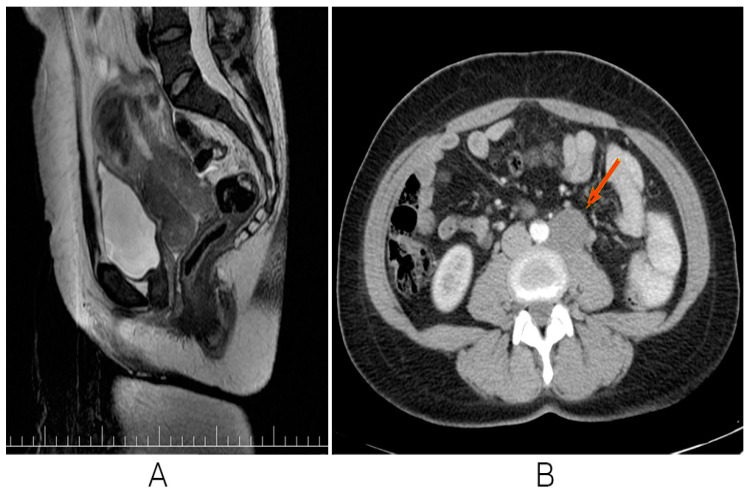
MRI SAG T2 sequence: cervical uterine hypointense lesion without gadolinium enhancement. (A) Contract CT. Arterial-phase retroperitoneal adenopathy (B).
